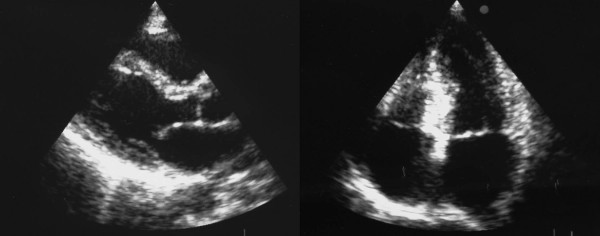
Transthoracic echocardiogram on day 5 after admission. Parasternal long-axis view (A) and apical 4-chamber view (B) showing recovery of wall-motion abnormalities with disappearance of apical ballooning.
